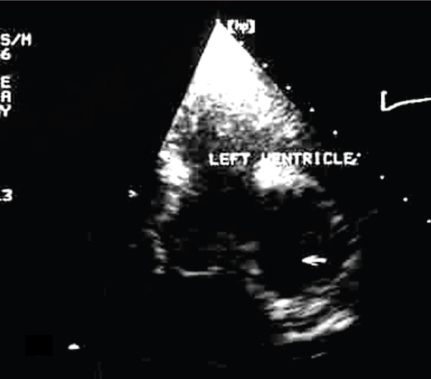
Apical four–chamber recording of the left ventricle and left atrium after surgery. An ovoid echo–free space (arrow)– the pericardial cavity after the tumor was removed.
